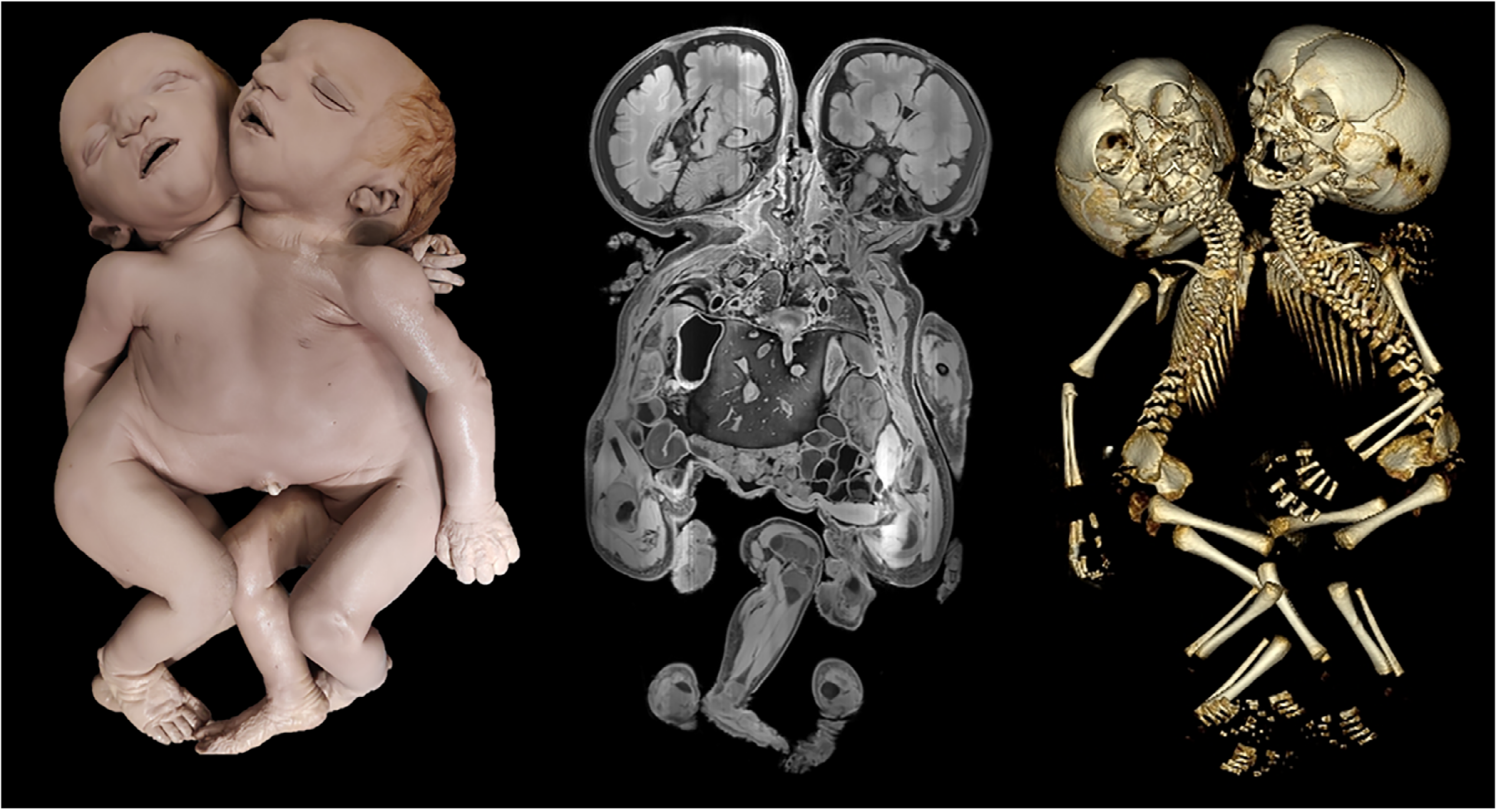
Example of radiological imaging of a conjoined twins from the anatomical museum in Nijmegen in which both magnetic resonance imaging (MRI) and computed tomography (CT) with three‐dimensional reconstructions were used to gain insight into its internal morphological characteristics
